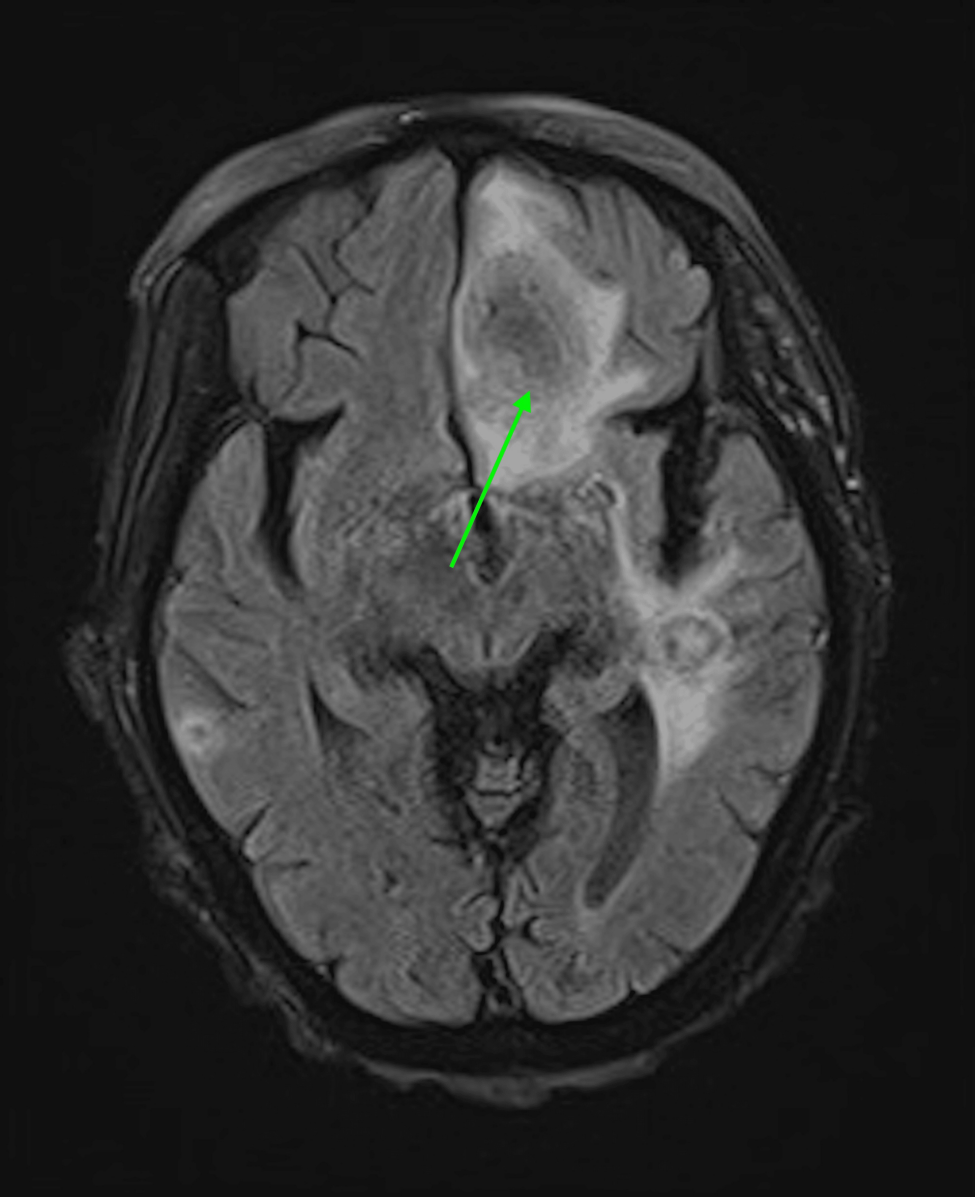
Initial brain MRI showing multiple ring-enhancing abscesses.
